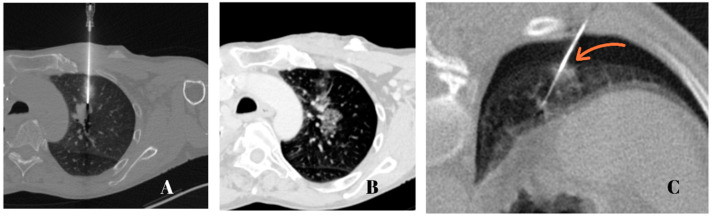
Axial CT images of the lungs at 75 mA, 120 kV, and a 2 mm slice thickness with a 1 mm increment: (A) A pulmonary mass is seen in the left upper lobe as the needle is entering the pleura at a 90° angle; (B) Post-intervention control scan showing a slight needle tract hemorrhage with no evidence of pneumothorax; (C) An intraprocedural pneumothorax as the needle enters the pleura at a very steep angle to target a pulmonary nodule (orange arrow) in the right lower lobe.
